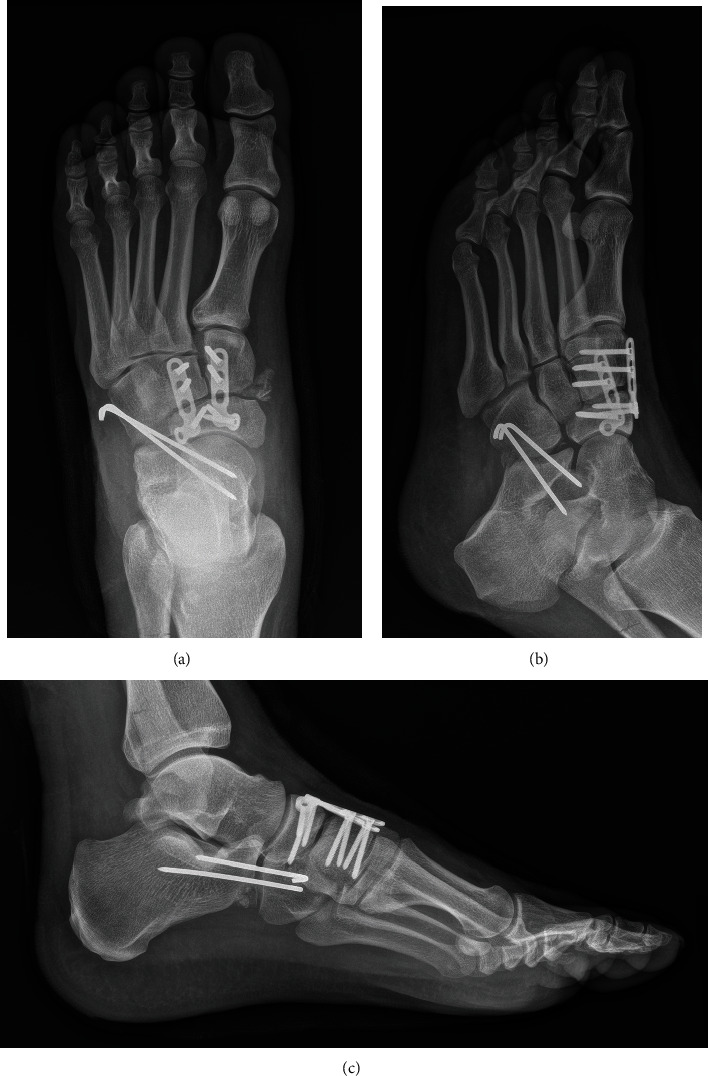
(a) Anteroposterior, (b) oblique, and (c) lateral X-rays of the left foot after open reduction, showing internal fixation of N-C1 and N-C2 joints, and pinning of the calcaneocuboid joint.
